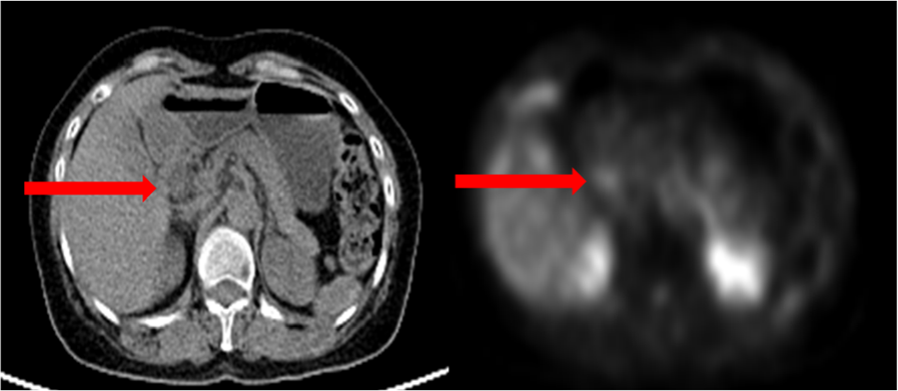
Positron emission tomography with integrated computed tomography image showing the hypermetabolic peripancreatic nodal relapse (*red arrow*)
